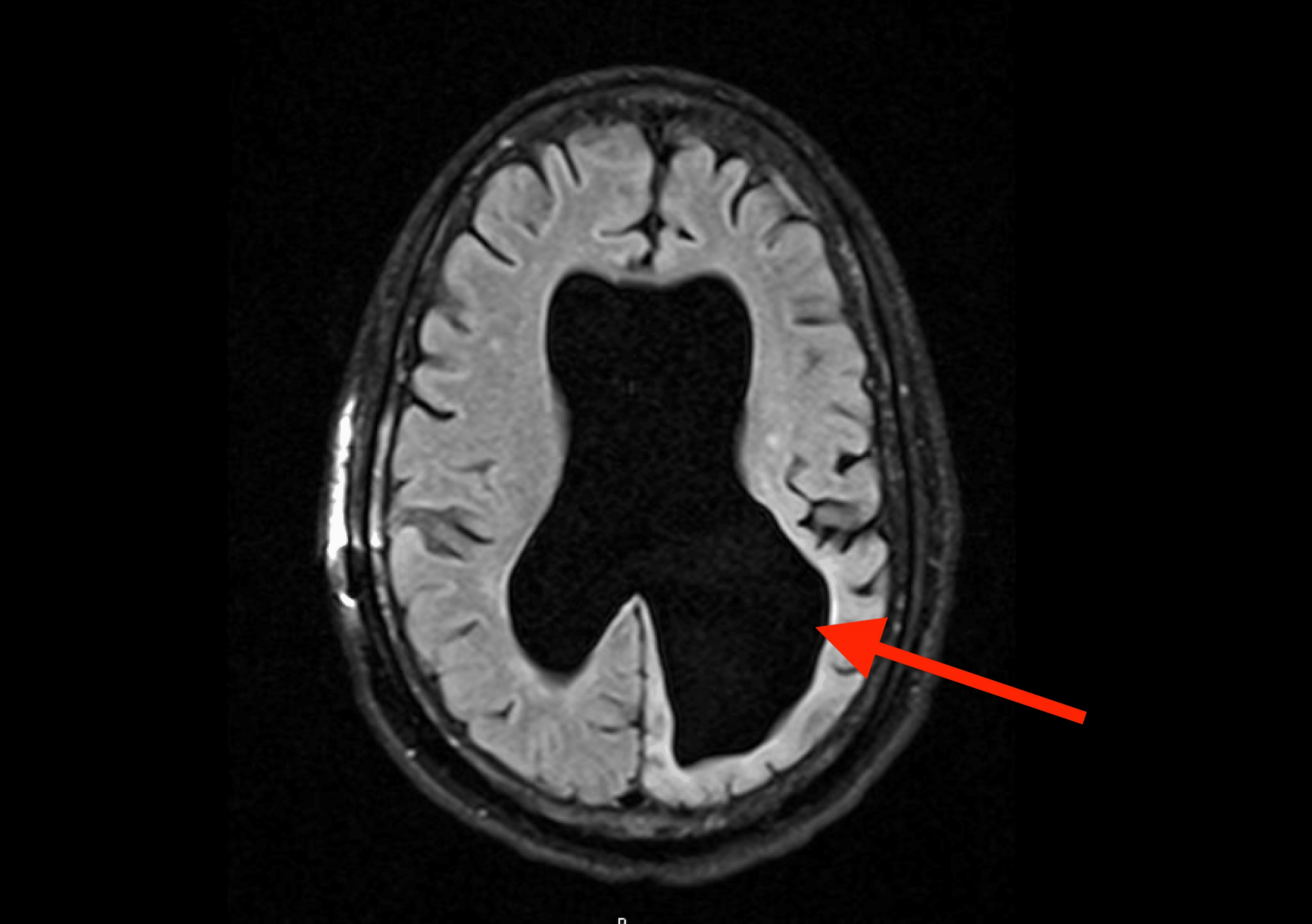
MRI brain showing stable biventricular dilatation and no evidence of leptomeningeal enhancement
